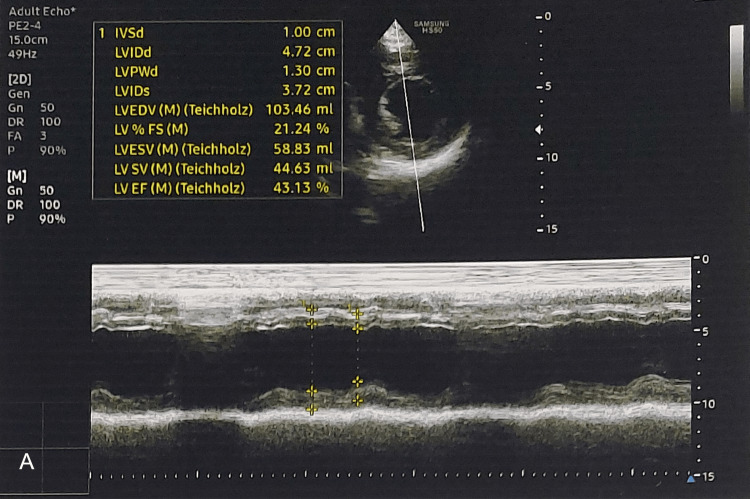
Echocardiography The echocardiography shows global hypokinesia of the left ventricle with moderate systolic dysfunction, mild mitral regurgitation, and an ejection fraction of 43%.
